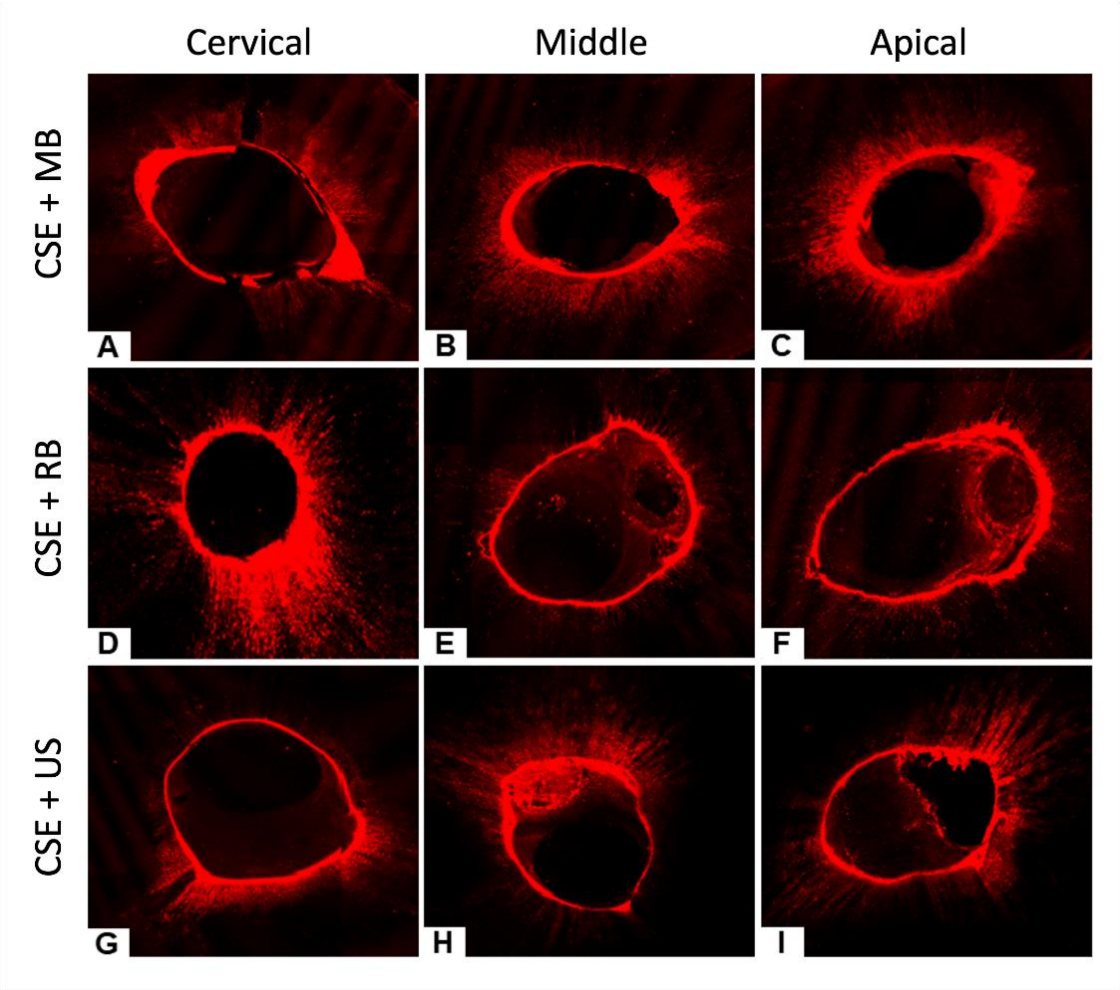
CLSM images showing the intratubular penetration of the Clearfil SE (CSE). Clearfil SE + microbrush cervical (A); middle (B); and apical third (C). Clearfil SE + rotary brush cervical (D); middle (E); and apical third (F). Clearfil SE + ultrasonic tip cervical (G); middle (H); and apical third (I).
